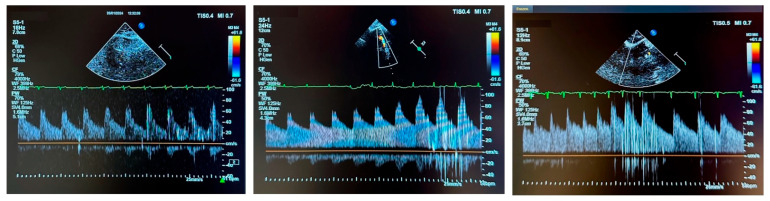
Examples of contrast-enhanced transcranial Doppler. Left Panel: ICC Category 2, Middle Panel: Category 3, Right Panel: Category 4.
